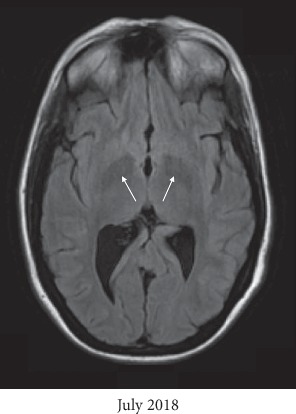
Supporting evidence for manganese neurotoxicity includes decreased signal intensity in the bilateral globus pallidi on axial T2-weighted images of the MRI brain from July 2018.
